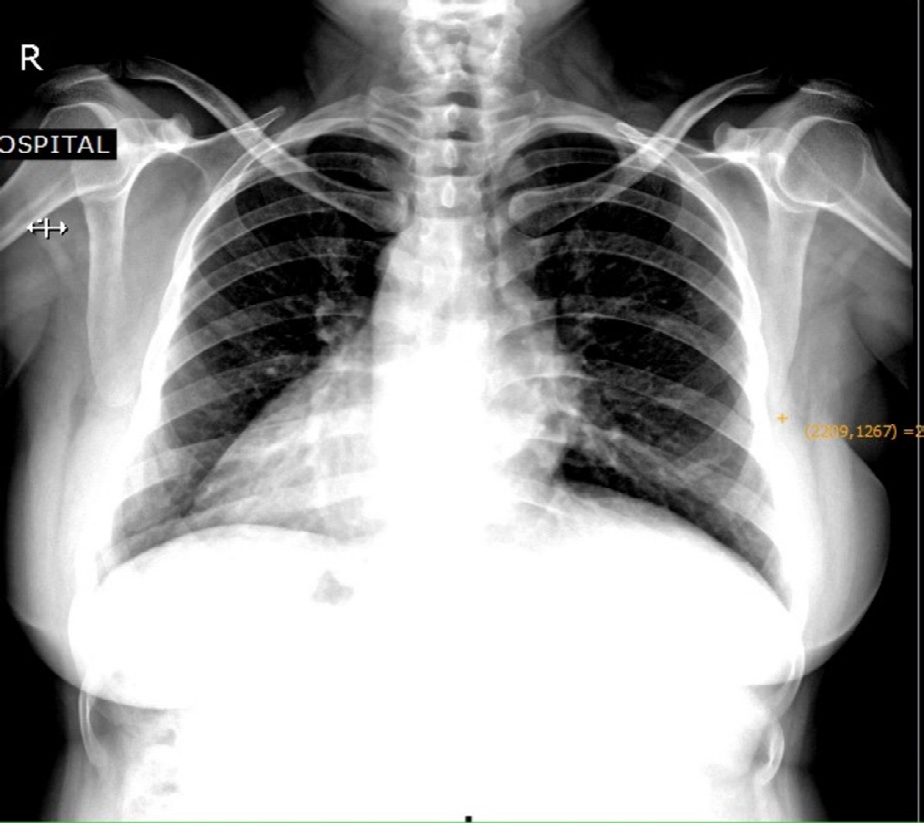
Chest X-ray showing dextrocardia.
